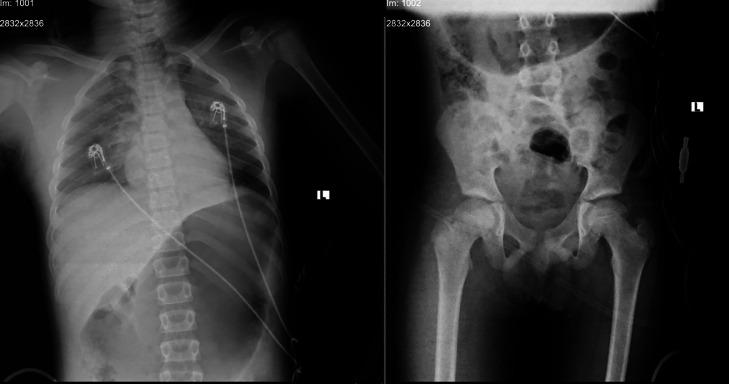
Chest and pelvic x-ray of the patient.
